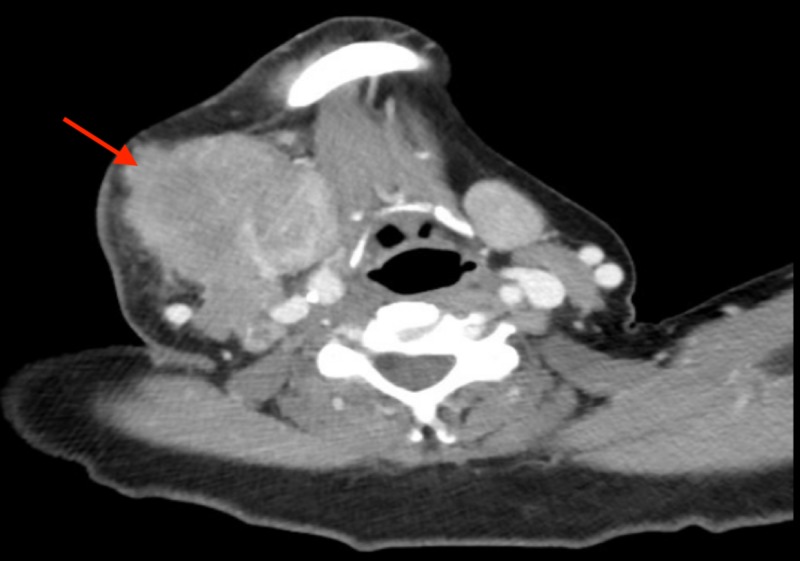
CT scan showing enlarging mass in the neck region.
